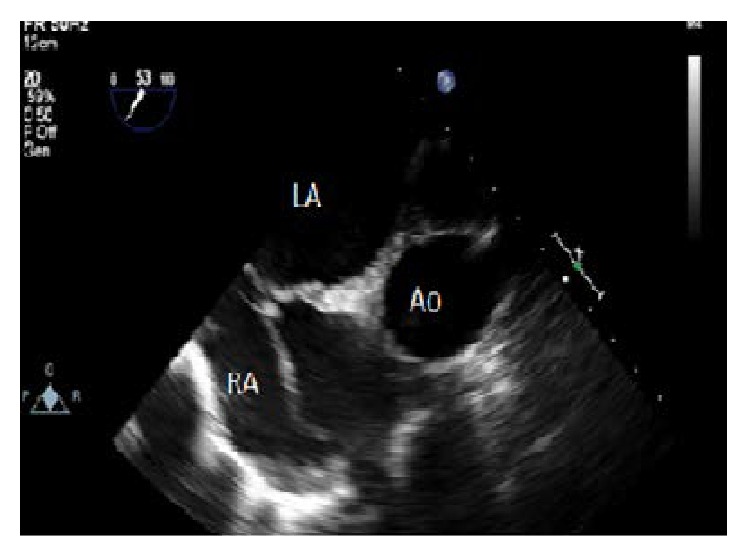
Thrombus formation in right atrium after the procedure, transesophageal echocardiography image of thrombus (Ao: Aorta, LA: Left Atrium, and RA: Right Atrium).
